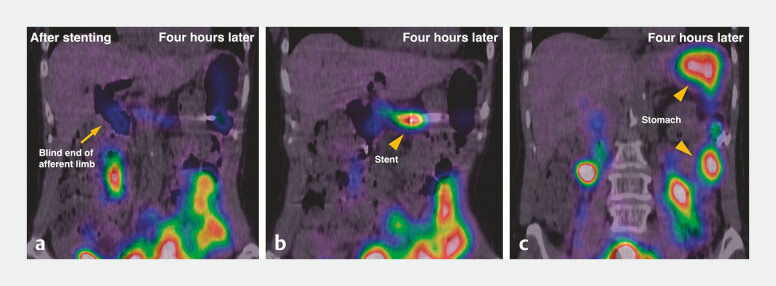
Hepatobiliary scintigraphy after stenting, on delayed imaging 4 hours later.
